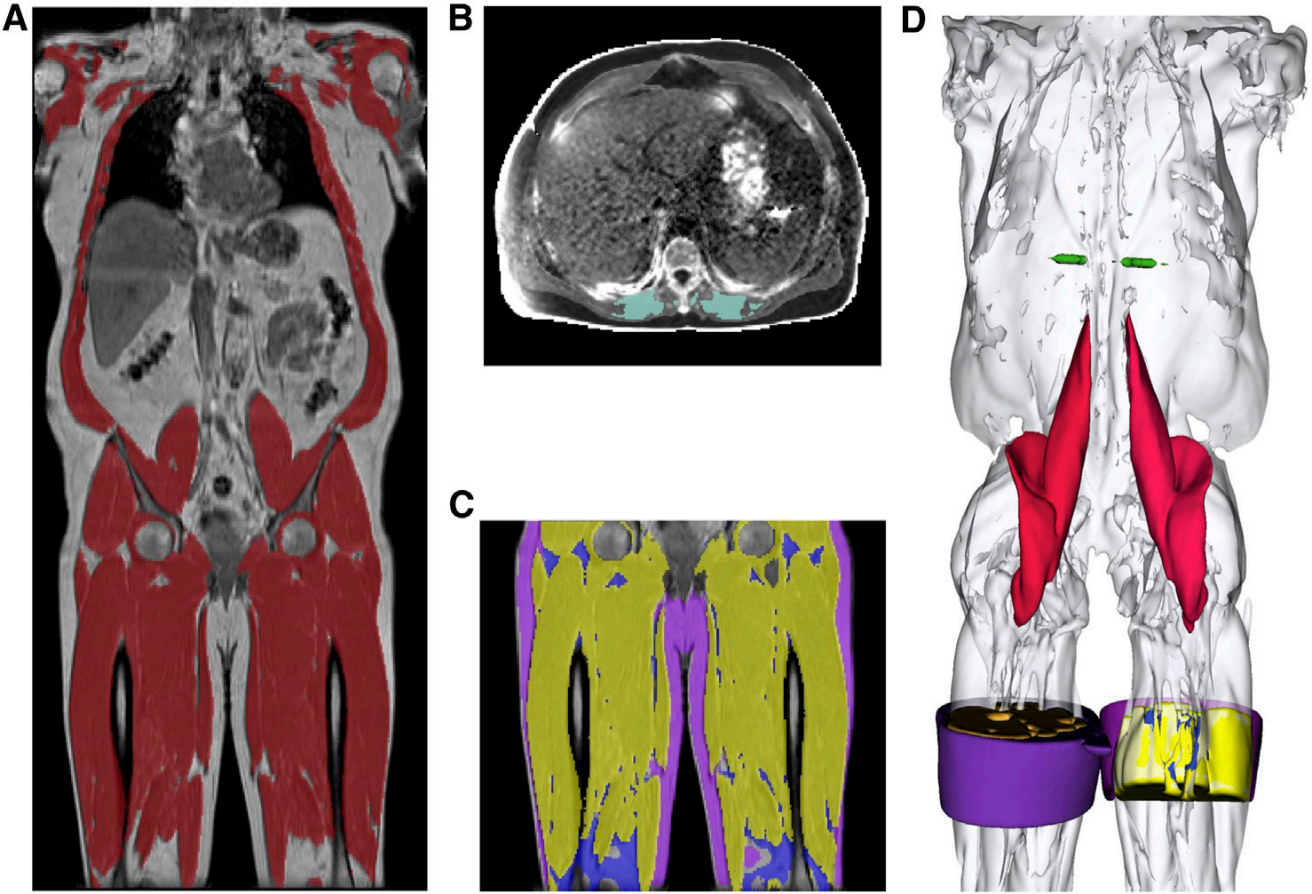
Segmentations from the UK Biobank abdominal MRI of a participant showing: (A) the total muscle (dark red) overlaid on the coronal view of a neck-to-knee scan, (B) the paraspinal muscle (green) in 2D quantitative MRI, (C) the thigh muscles (yellow), thigh IMAT (blue), thigh SAT (purple) overlaid on the coronal view, and (D) 3D renderings of the muscle segmentations for the following structures: mid-thigh muscle (yellow), mid-thigh IMAT (blue), mid-thigh subcutaneous adipose tissue (purple), paraspinal muscles (green), iliopsoas muscles (red) and total muscle (white).
